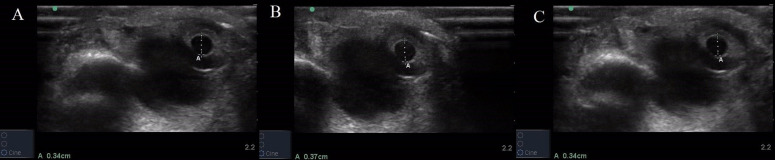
PD assessment in closed eye by B-mode ultrasound. Crosses represent the markers set by the examiner for measuring PD. A, PD at rest. B, PD on direct light stimulation. C, PD on consensual light stimulation. PD, pupillary diameter.
